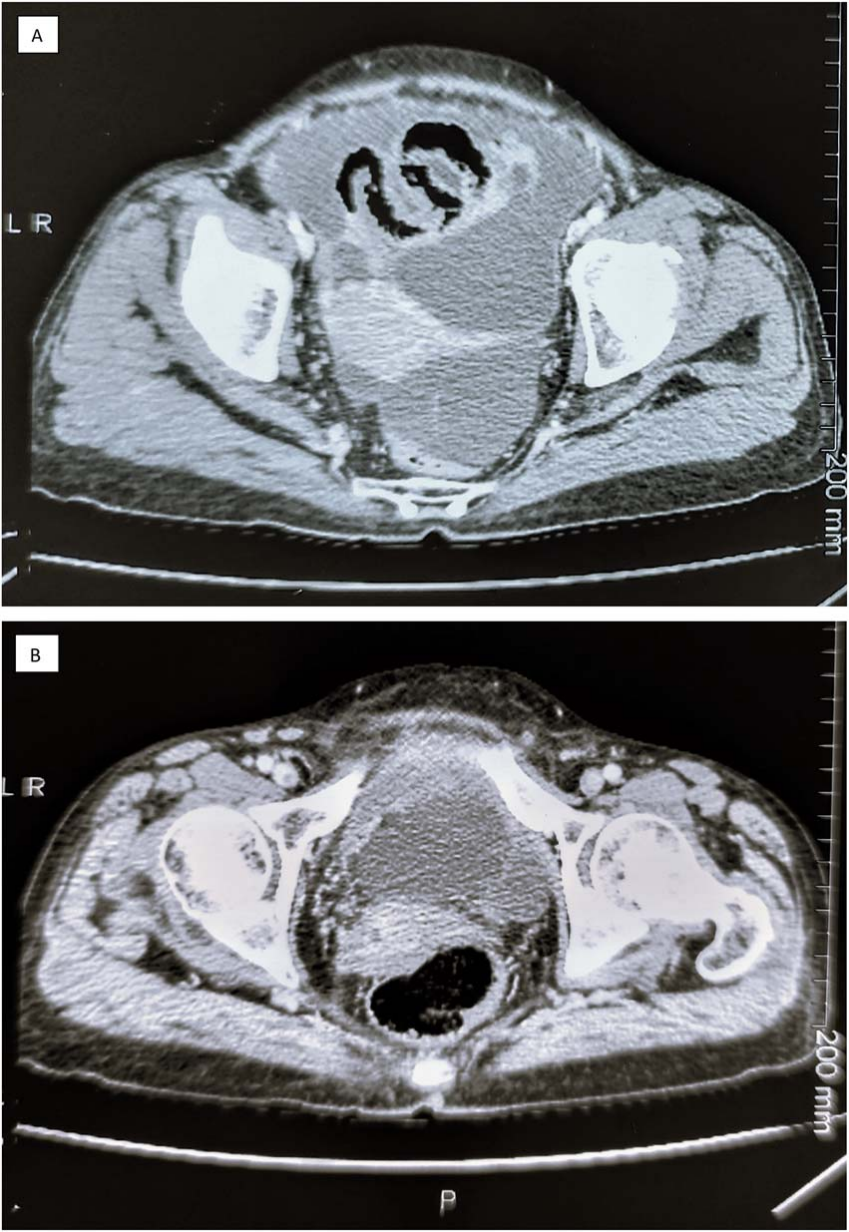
The Computed tomography of the abdomen and pelvis showing a massive ascites (A) with bilateral latero-uterine heterogeneous masses and peritoneal carcinomatosis (B).
